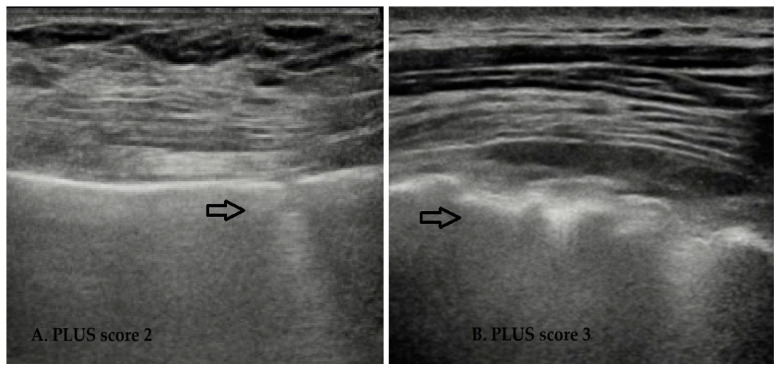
Pleural fragmentation (score 2, from 0 to 3, of pleural abnormalities) (arrow (A)) and consolidation (score 3, from 0 to 3, of pleural abnormalities) (arrow (B)) in RA patients upon lung ultrasound examination.
